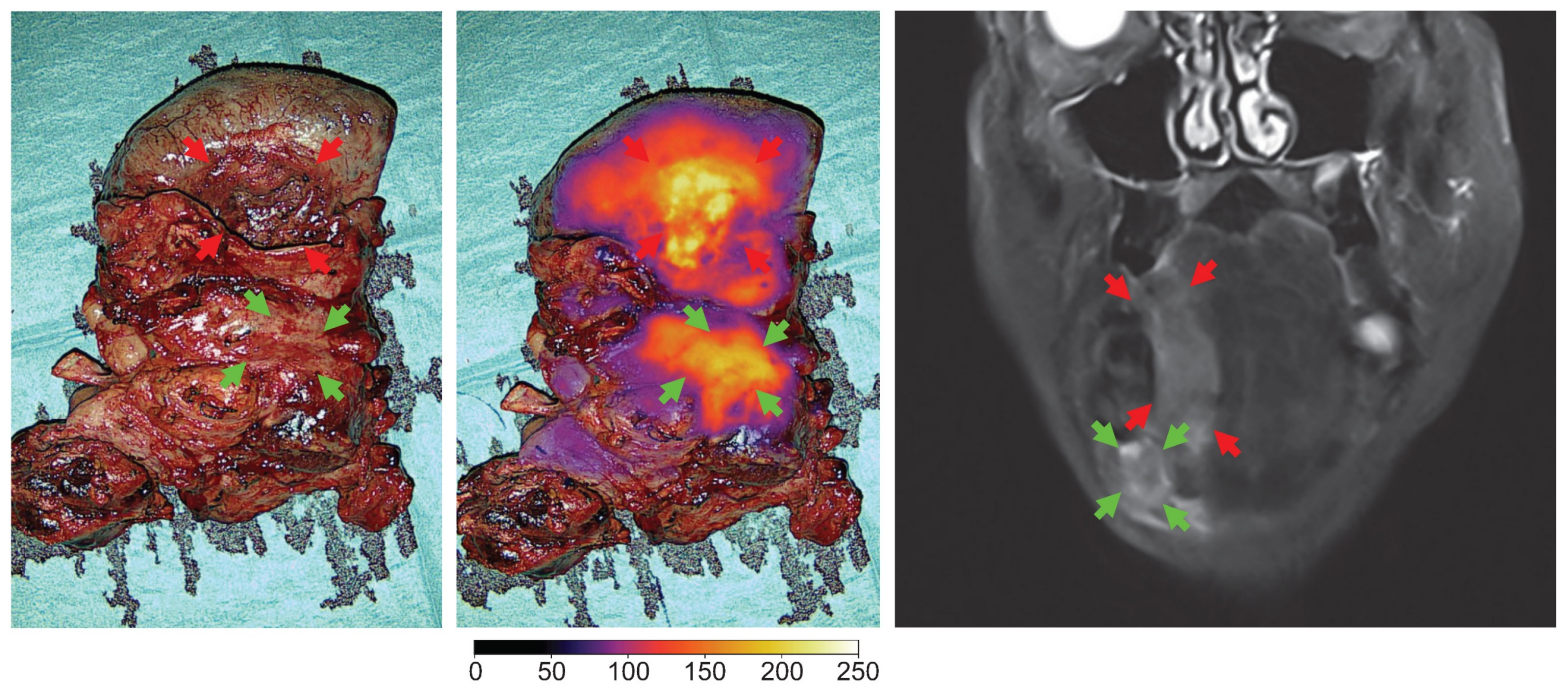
Optical imaging of the en bloc resection of a FOM/lateral tongue cancer with regional metastasis (level II) in white light and overlay, and the preoperative MRI of the tumor (case 7). Red arrows: tumor, green arrows: neck metastasis. Gain: 1%.
